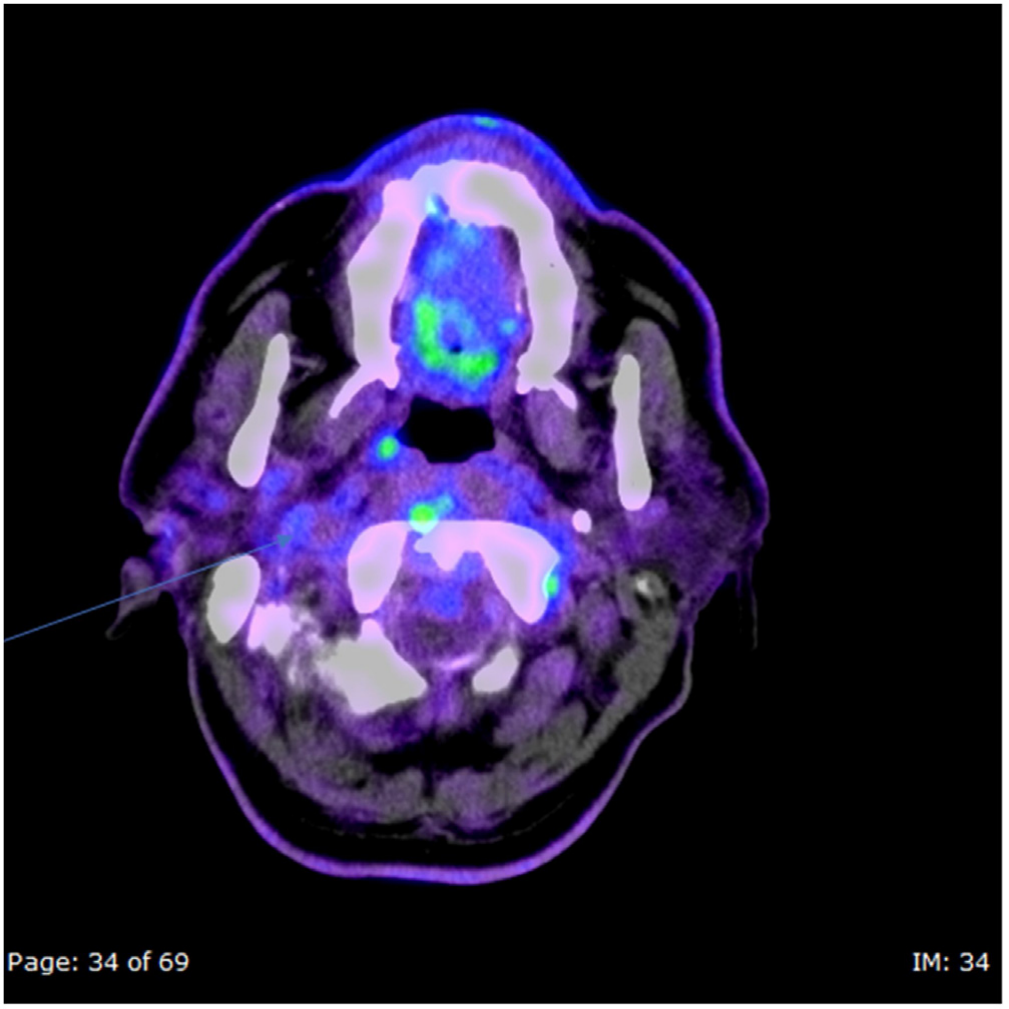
Axial FDG PET-CT demonstrates mild increased avidity in the right deep neck spaces including carotid and masticator spaces (arrow). The “low grade” nature of the tracer uptake suggests infective cause rather than malignant.
